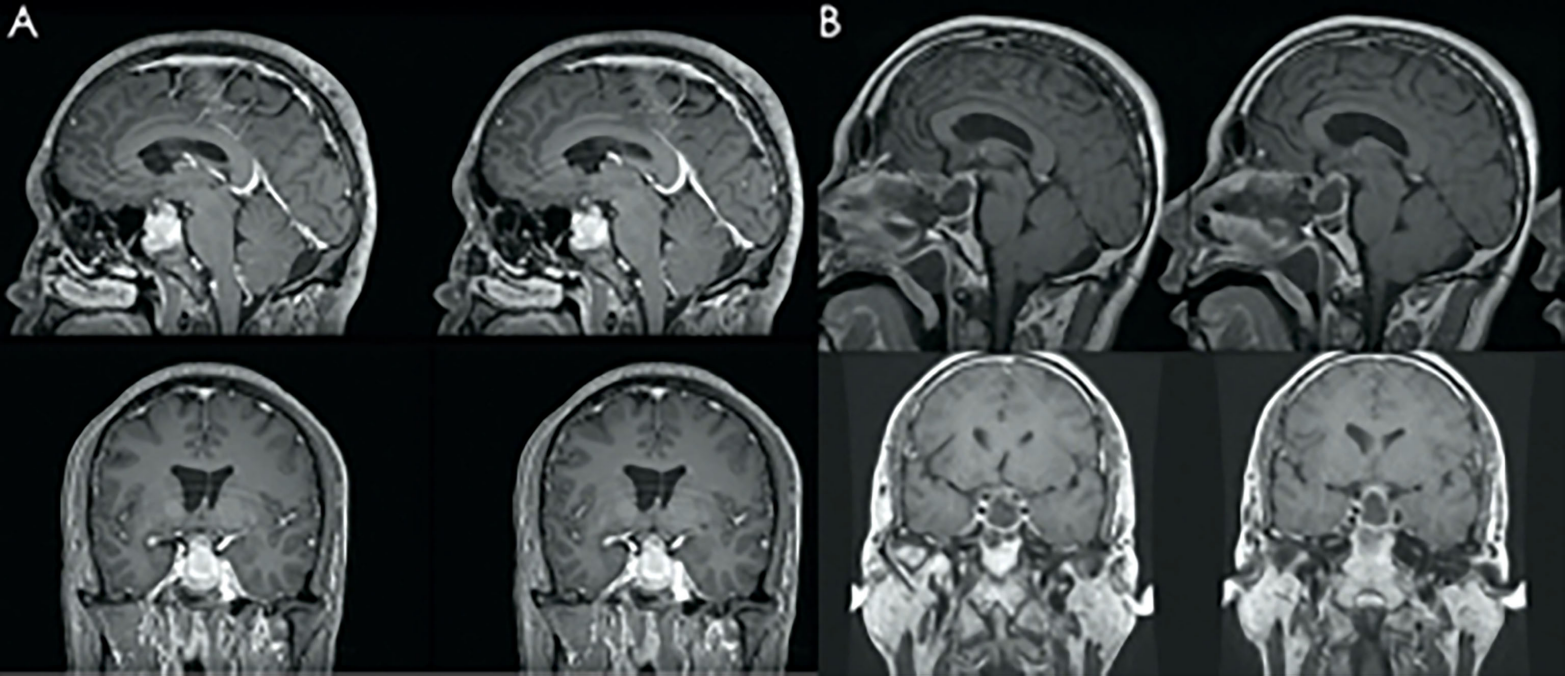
Representative image of preoperative magnetic resonance imaging (MRI) (A) and postoperative MRI follow-up (B). The MRI shows gross total resection of the tumor.
